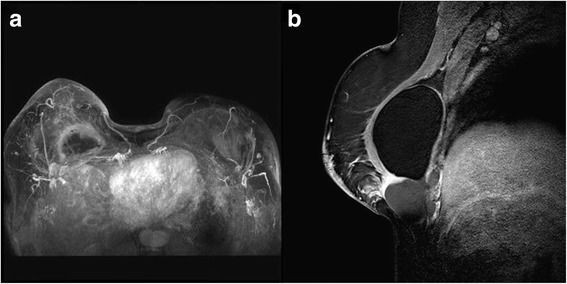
Magnetic resonance imaging showing a mass in the lower inner quadrant of the right breast with rim contrast-enhancement abutting the implant surface. a Sagittal fat suppressed contrast-enhanced T1-weighted image. b Maximum intensity projection image
